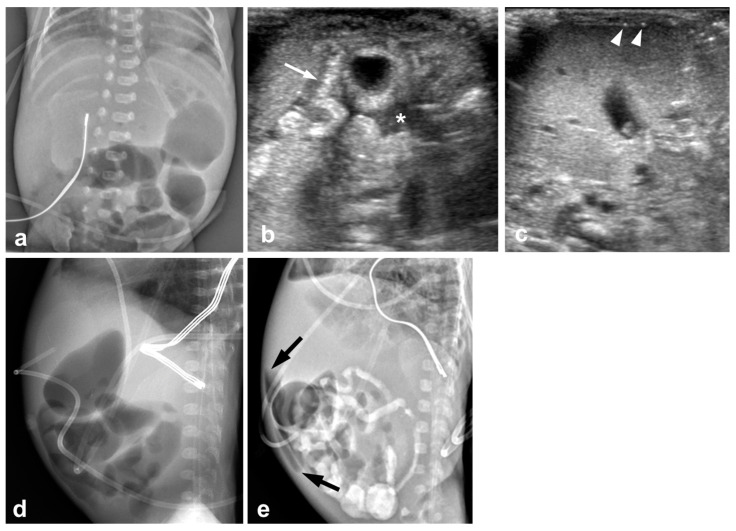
12-day-old boy (born at gestational age of 24 weeks and 1 day) with perforated necrotizing enterocolitis and radiographic pneumoperitoneum; this infant died 11 days after laparotomy. (a) An abdominal radiograph obtained 1 day before US shows nonspecific distension of the bowels without free air. (b,c) Preoperative US images show increased intestinal wall echogenicity and wall thickening (arrow), complex ascites (asterisk), and free air (arrowheads) between the hepatic surface and the anterior abdominal wall. (d) Preoperative abdominal radiographs obtained 1 h after US show no pneumoperitoneum. (e) Pneumoperitoneum (arrows) appears on abdominal radiographs obtained 35 h after US.
